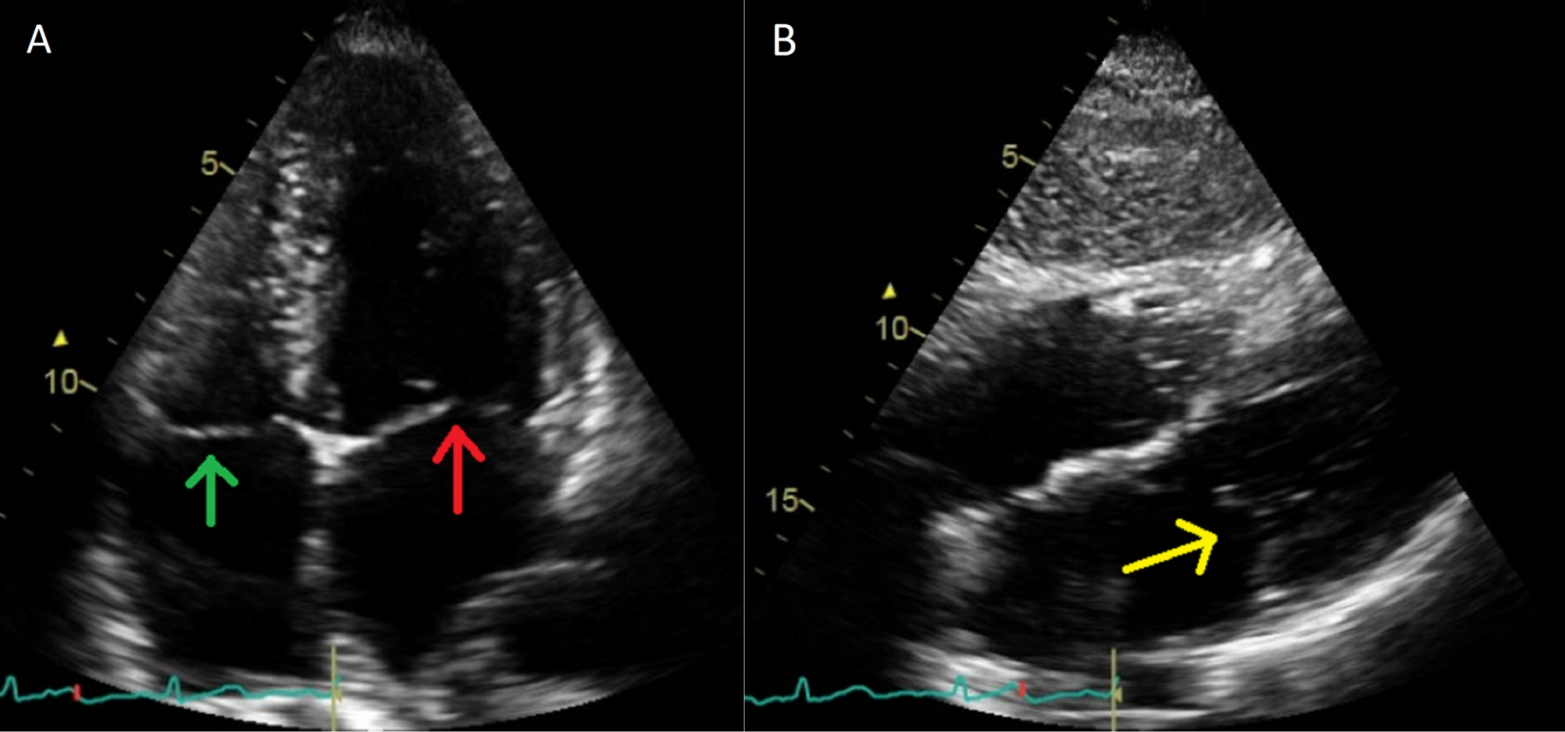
TTE images of the patient A: four chamber TTE; B; aortic longitudinal TTE. The images show no vegetations or abscesses involving any of the valves Green arrow: tricuspid valve; red arrow: mitral valve; yellow arrow: aortic valve TTE: transthoracic echocardiogram
